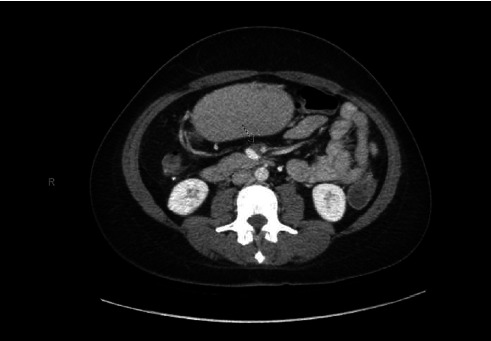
CT scan of the abdomen and pelvis revealing a 3-cm central mesenteric mass invading the duodenum, encasing the superior mesenteric artery (SMA), and abutting the superior mesenteric vein (SMV). A new 8-mm lesion in the peripheral hepatic segment 5/8 is also identified (indicated by the arrow).
